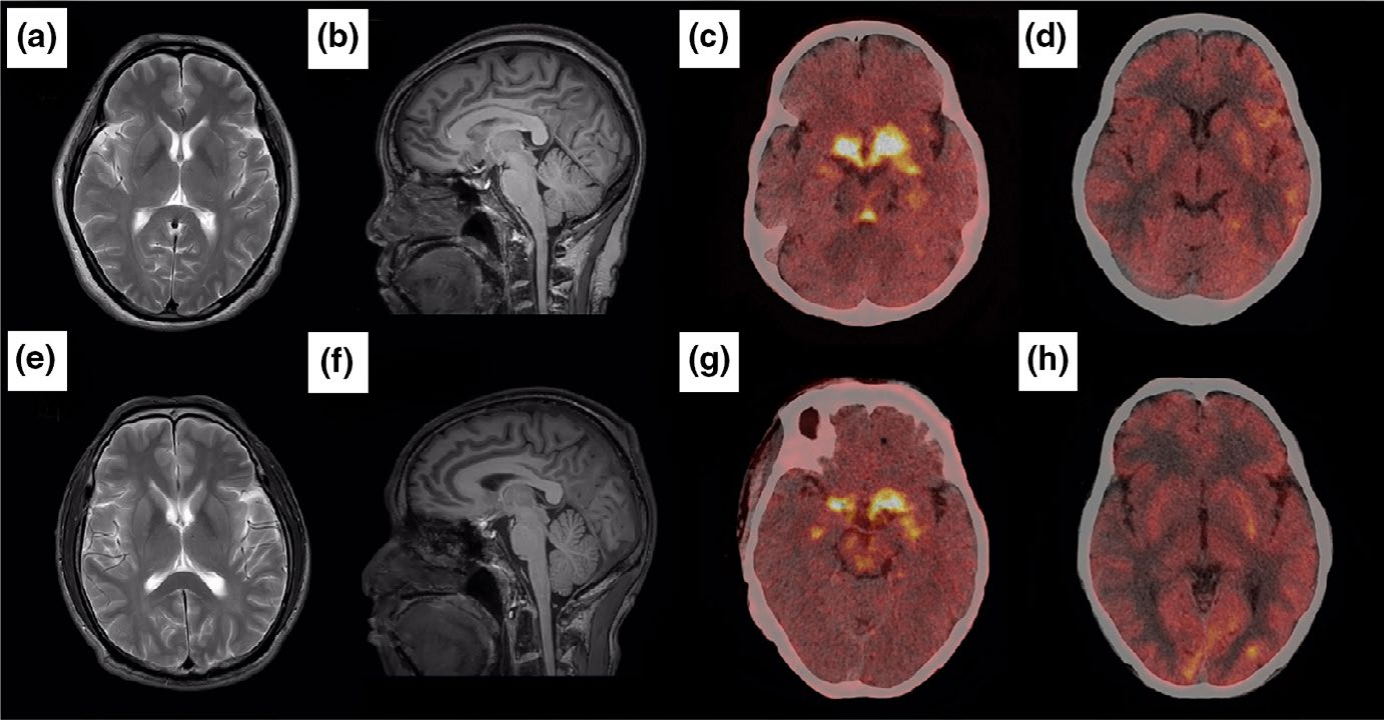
Images of the brains of II‐3 (a‐d) and II‐4 (e‐h) obtained using 3.0T magnetic resonance imaging (MRI), ^18^F‐DOPA, and ^18^F‐FDG positron emission computed tomography imaging (PET/CT)
